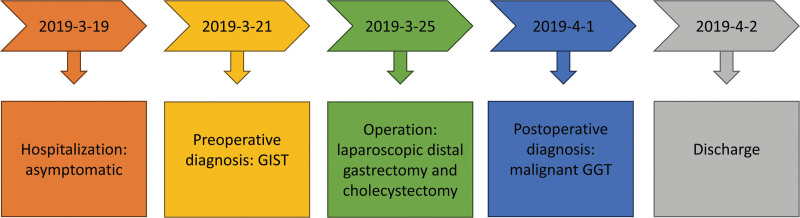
A timeline of this clinical case: the preoperative diagnosis was made by enhanced abdominal CT and ultrasound gastroscopy, and postoperative diagnosis was made by postoperative pathology.
